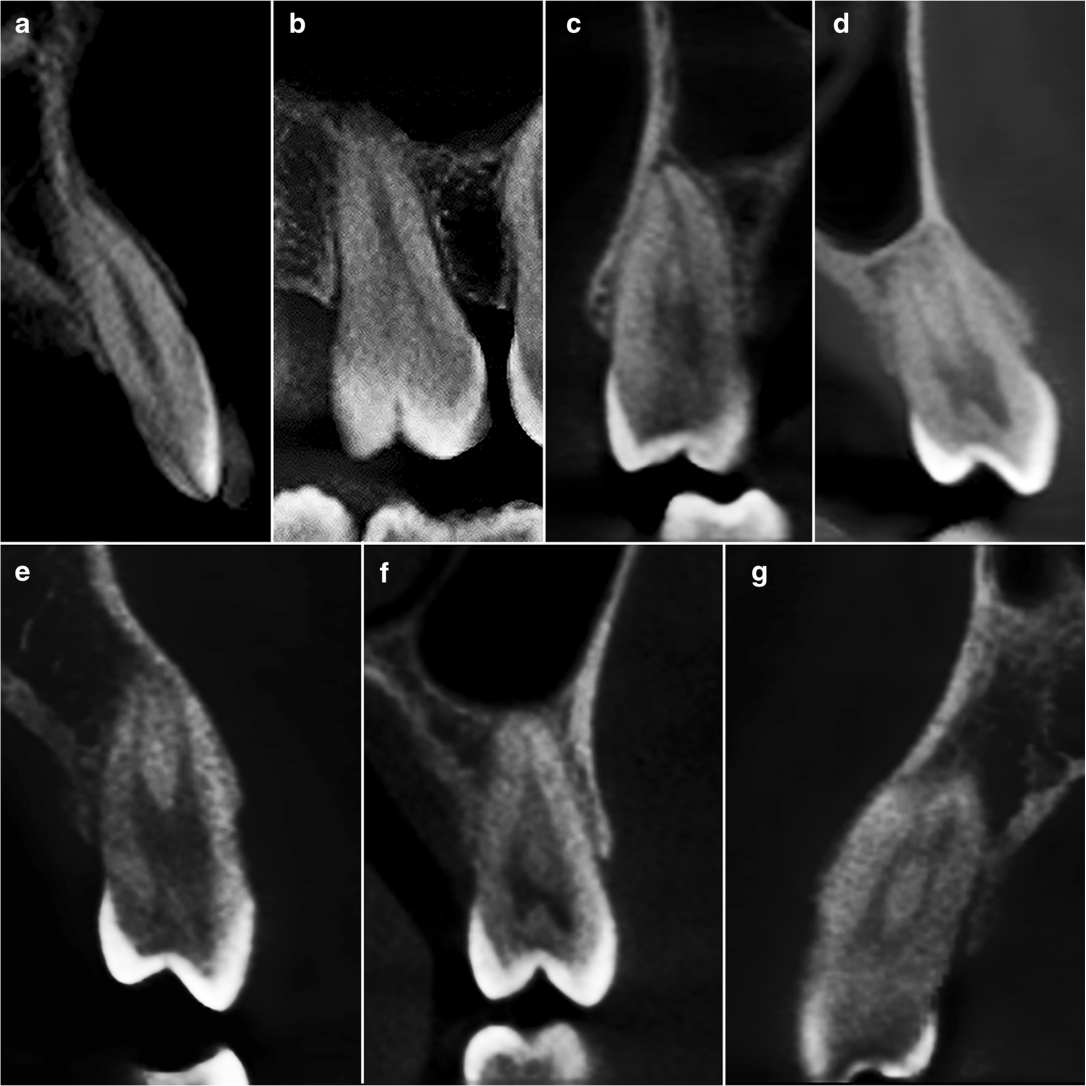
CBCT images of maxillary teeth in custom planes (reconstructed based on axial, sagittal and coronal planes) based on Vertucci’s classification. a Type I – right maxillary central incisor (b) Type II – right maxillary first molar (c) Type III – right maxillary second premolar (d) Type IV – left maxillary first premolar (e) Type V – left maxillary first premolar (f) Type VI – left maxillary second premolar (g) Type VII – right maxillary first premolar
